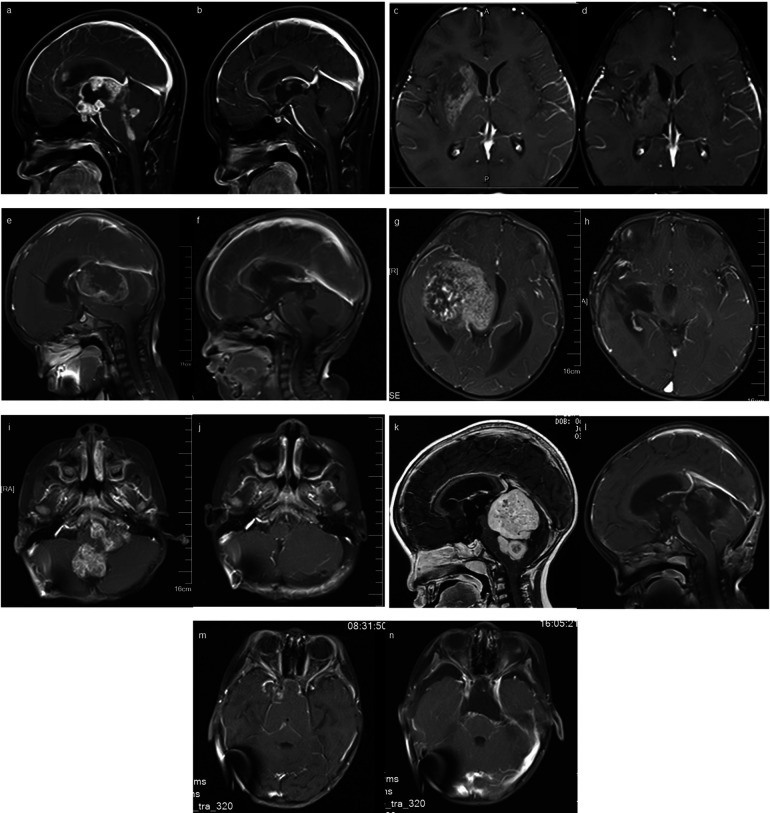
The typical preoperative and follow-up MRI images of several patients. a and b: CNS germinoma metastasis, before and after “chemotherapy and RT”; c and d: CNS germinoma in basal ganglia, before and after “chemotherapy and RT”; e and f: pinealoblastoma, before and after “operation + chemotherapy and RT”; g and h: high-grade malignant astrocytoma, before and after “operation + chemotherapy and RT”; i and j: ependymoma, before and after “operation + RT”; k and l: medulloblastoma, before and after “operation + chemotherapy and RT”; m and n: craniopharygioma, before and after “operation”;.
